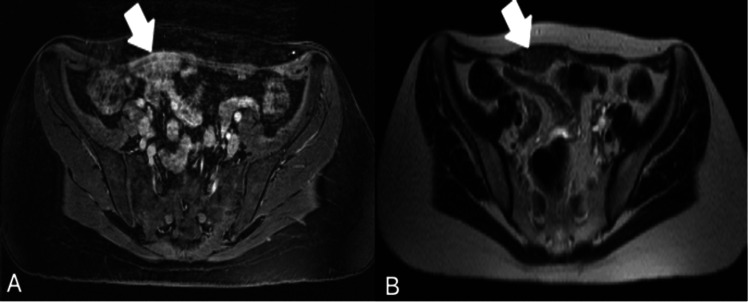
An MRI scan of the abdomen with intravenous contrast Panel A: T1 post-contrast fat-saturated weighted image depicts a lesion with avid enhancement in the right rectus abdominis muscle (white arrow). Panel B: A T2-weighted image reveals a lesion with higher signal intensity in the right rectus abdominis muscle compared to the left (white arrow).
